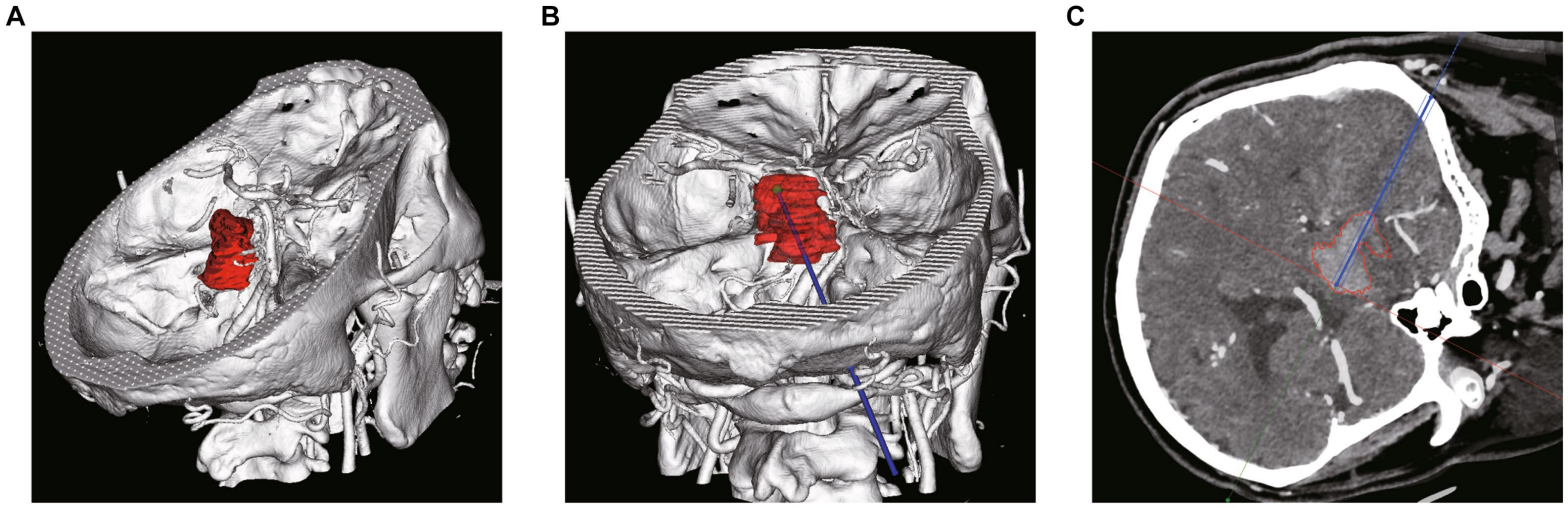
Pre-operative plan using robot planning system. (A) 3D shape and location reconstruction of hematoma. (B) The target and drainage path was planned through the long axis of the hematoma. (C) Avoiding the dentate gyrus and blood vessels on the basis of CTA and CT image fusion.
